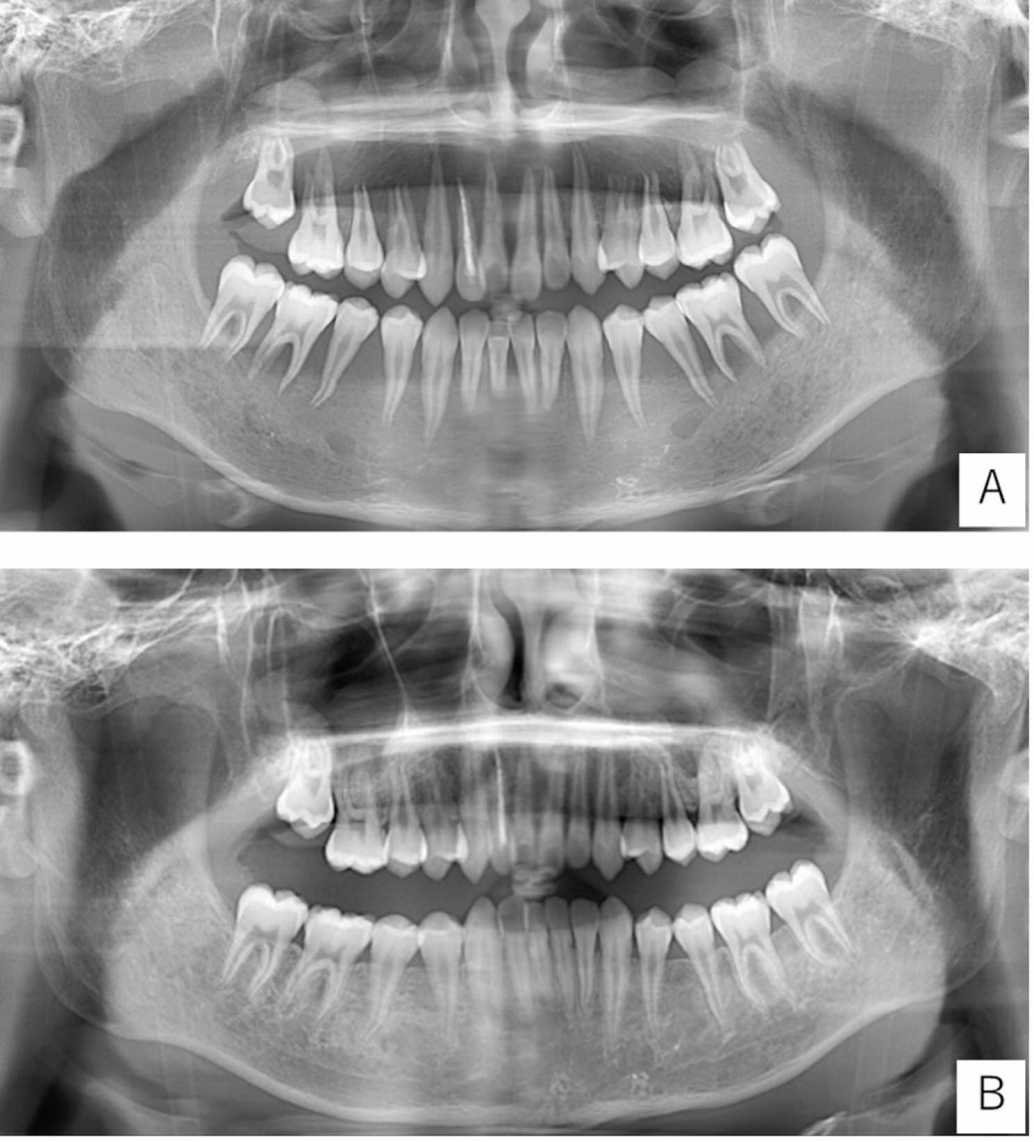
Panoramic radiographs. A Before treatment. B 116 days after the initiation of chemotherapy
